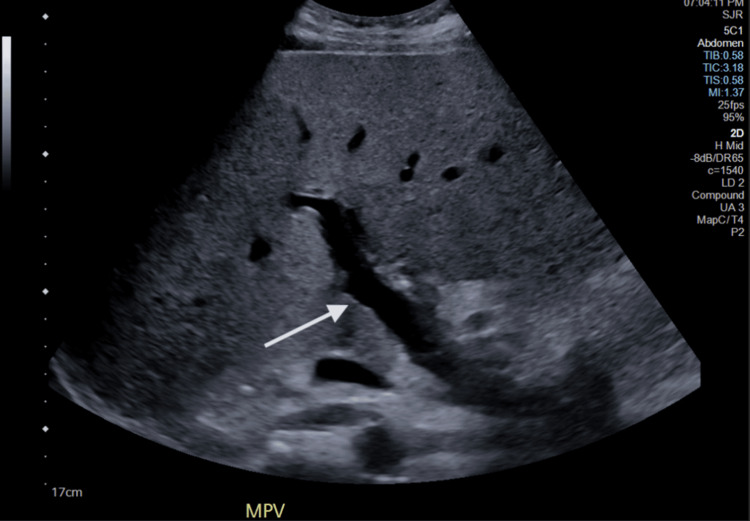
Abdominal ultrasound demonstrating patent main portal vein without thrombosis or flow obstruction (white arrow). Hepatic parenchyma demonstrated increased echogenicity consistent with steatosis. The absence of macrovascular occlusion supports microvascular ischemic hepatopathy as the mechanism of liver injury.
